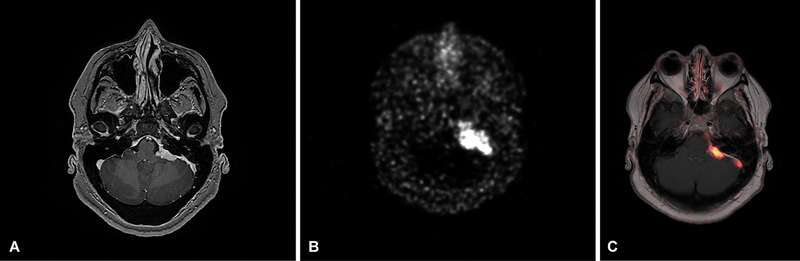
Axial T1-weighted post-contrast image of a left posterior fossa extra-axial dural-based lesion (
